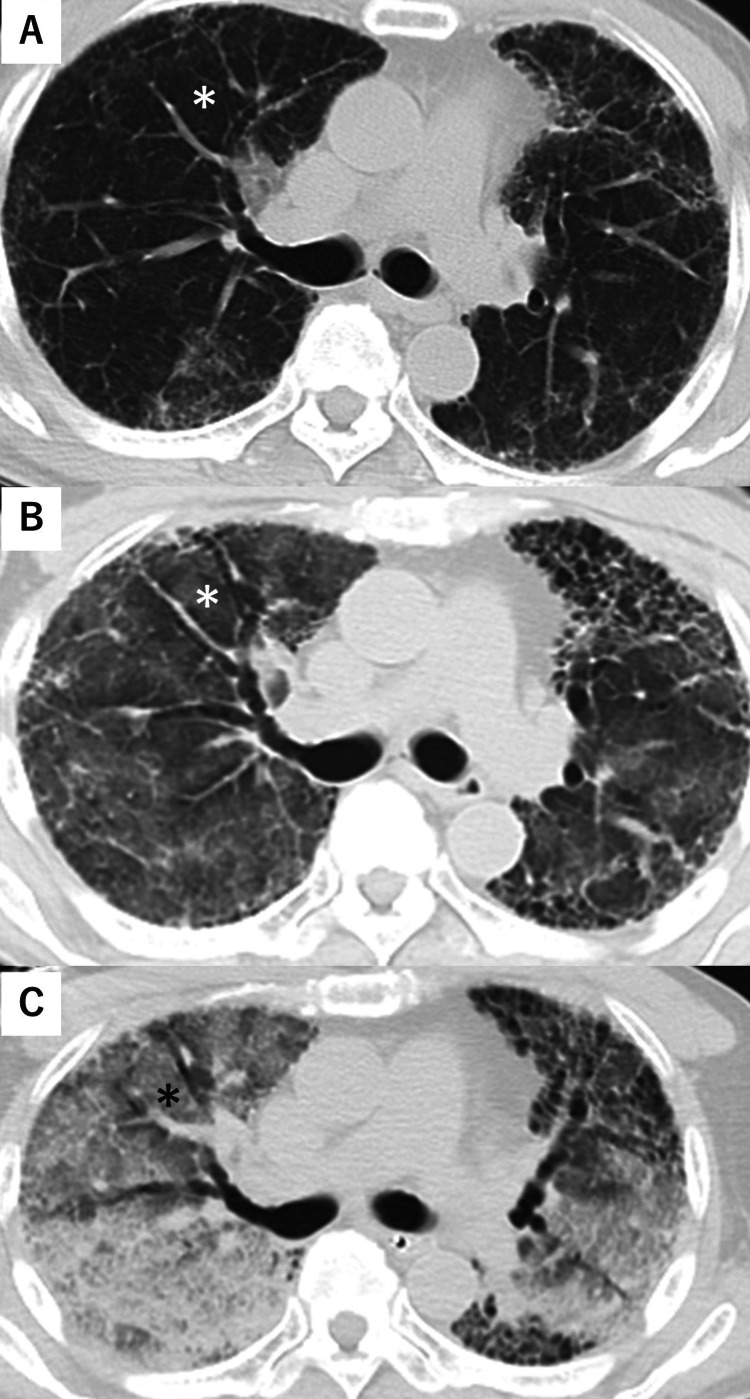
Chest computed tomography (CT) (upper [A]: 4 years before; middle [B]: referral hospital; lower [C]: on arrival) On the CT scan from four years ago, the ground-glass opacity is not clearly defined (A). At the referral hospital, diffuse ground-glass opacities appeared (B). After transfer to our hospital, the ground-glass opacity has worsened (C). Focusing on the right segment 3 (*), the difference is evident.
